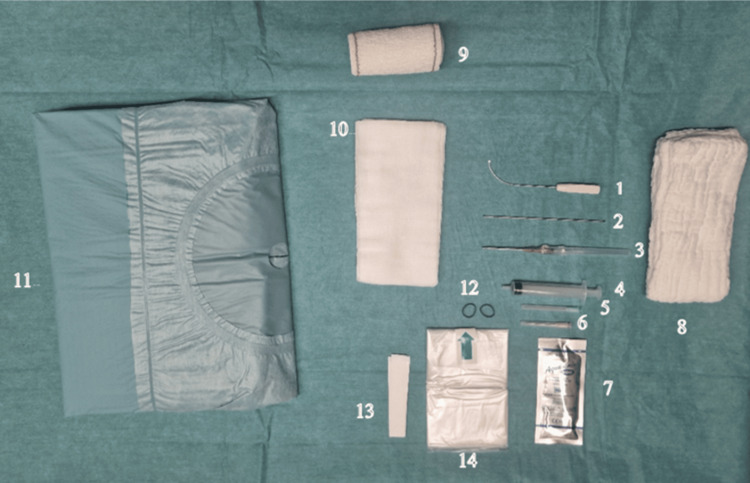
Material needed to perform the surgery. The probe cover (14) was attached to the probe with elastic rubber bands (12) and fixed on the sterile drape with tapes (13). A sterile ultrasound gel (7) is applied between the probe cover and the patient skin. The patient is positioned in the supine position with the operated hand placed on a table and stabilized with two abdominal sponges (8) in the neutral position of the wrist. An 18G needle (6) is used to harvest the lidocaine in a 10cc syringe (4) and a 20 G needle (5) for injection through the entry point. A 14G catheter (3) is then introduced through the skin and the forearm fascia to increase the entry point size and replaced by the round-ended stylus (2) to palpate the TCL and define the cut trajectory.  After the stylus removal, the cutting instrument (1) is introduced through the entry point to perform the antegrade release. After the release, the round-ended stylus is used to palpate the ligament and be sure of the completeness of its release. If this is not the case, the exact location of the remaining fibers is defined by probe palpation, and the cutting instrument is re-introduced to complete the release. Three 10x20 sponges (10) are then used to make a compressive dressing with a Velpeau band (9) that will be removed by the patient after 12 hours.
